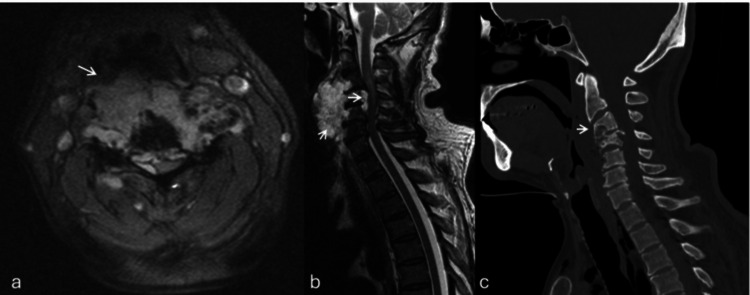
Preoperative imaging of a cervical chordoma with extensive bone and soft tissue involvement. (a) Axial T2-weighted fat-suppressed MRI (STIR sequence) reveals a heterogeneously hyperintense mass involving the cervical vertebrae with marked infiltration of the prevertebral and paravertebral soft tissues (arrow). (b) Sagittal T2-weighted MRI shows destruction of the mid-cervical vertebral bodies and significant spinal cord compression (arrows). (c) Sagittal CT in the bone window demonstrates lytic vertebral body destruction with collapse and structural compromise of the cervical spine (arrow). MRI: magnetic resonance imaging, STIR: short tau inversion recovery, CT: computed tomography
